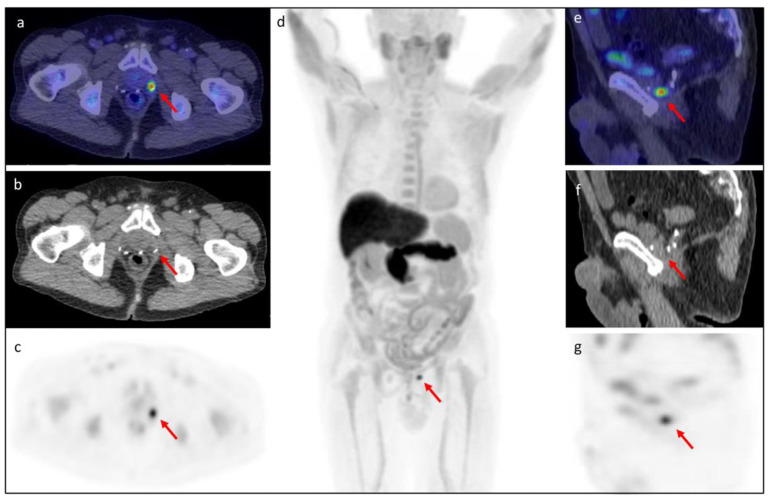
[^18^F]fluciclovine PET/CT performed in a 75-year-old patient with BCR after radical prostatectomy (Gleason Score 7 = 4 + 3). At the time of the PET/CT, PSA rose to 0.89 ng/mL with PSAdt of 7.8 months. The (d) MIP, (a–c) axial (a: fused PET/CT, b: CT, c: PET) and (e–g) sagittal (e: fused PET/CT, f: CT, g: PET) images showed a locoregional recurrent lesion in left prostatectomy bed (red arrow) with SUVmax 8.6.
